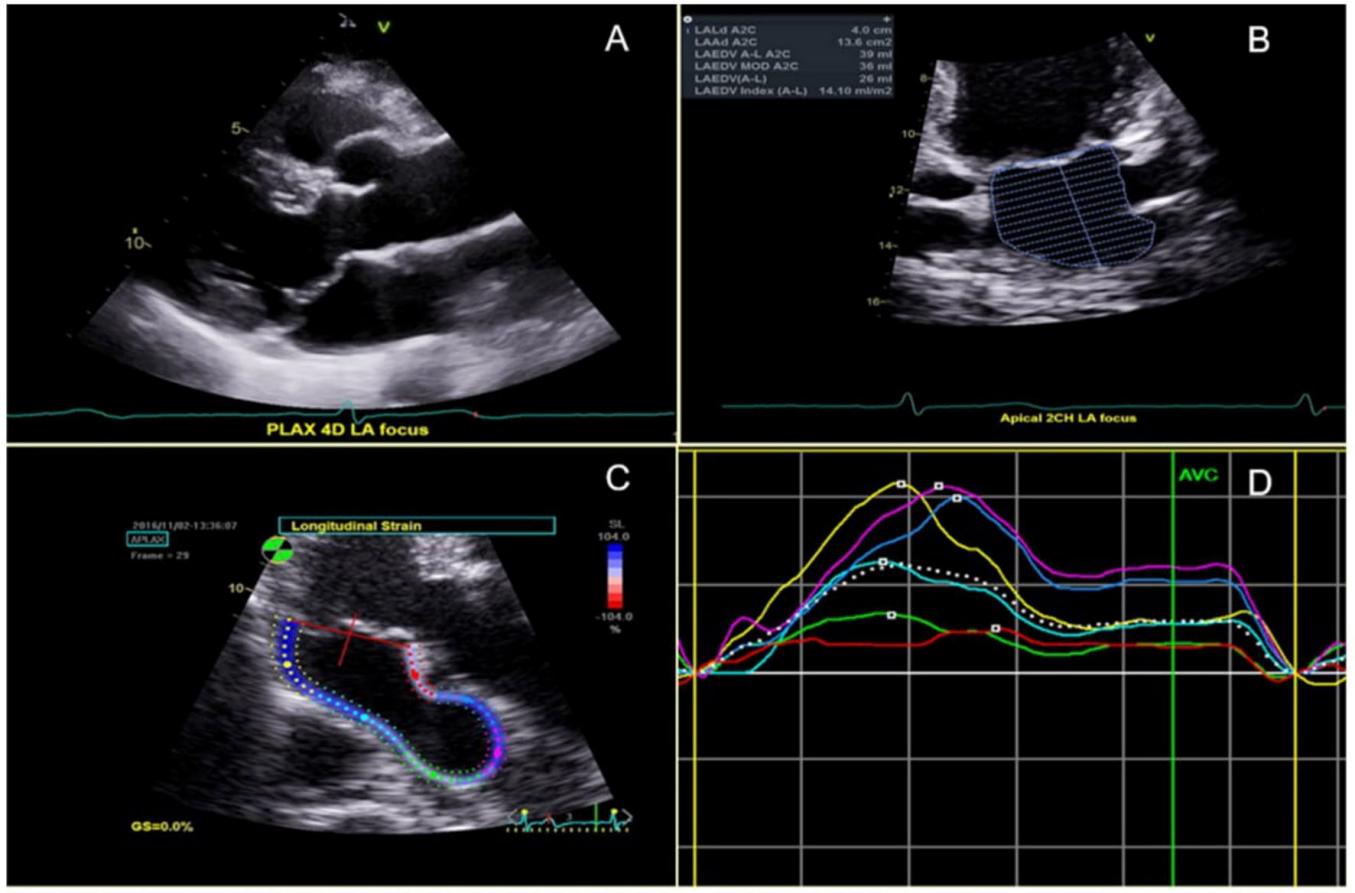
(A) Bileaflet MVP shown in the parasternal long axis view. (B) Left atrial (LA) end-diastolic volume in the 2-chamber view. (C) 2D-Segmental LA strain from a zoomed apical long axis view; and (D) corresponding segmental LA strain graph.
